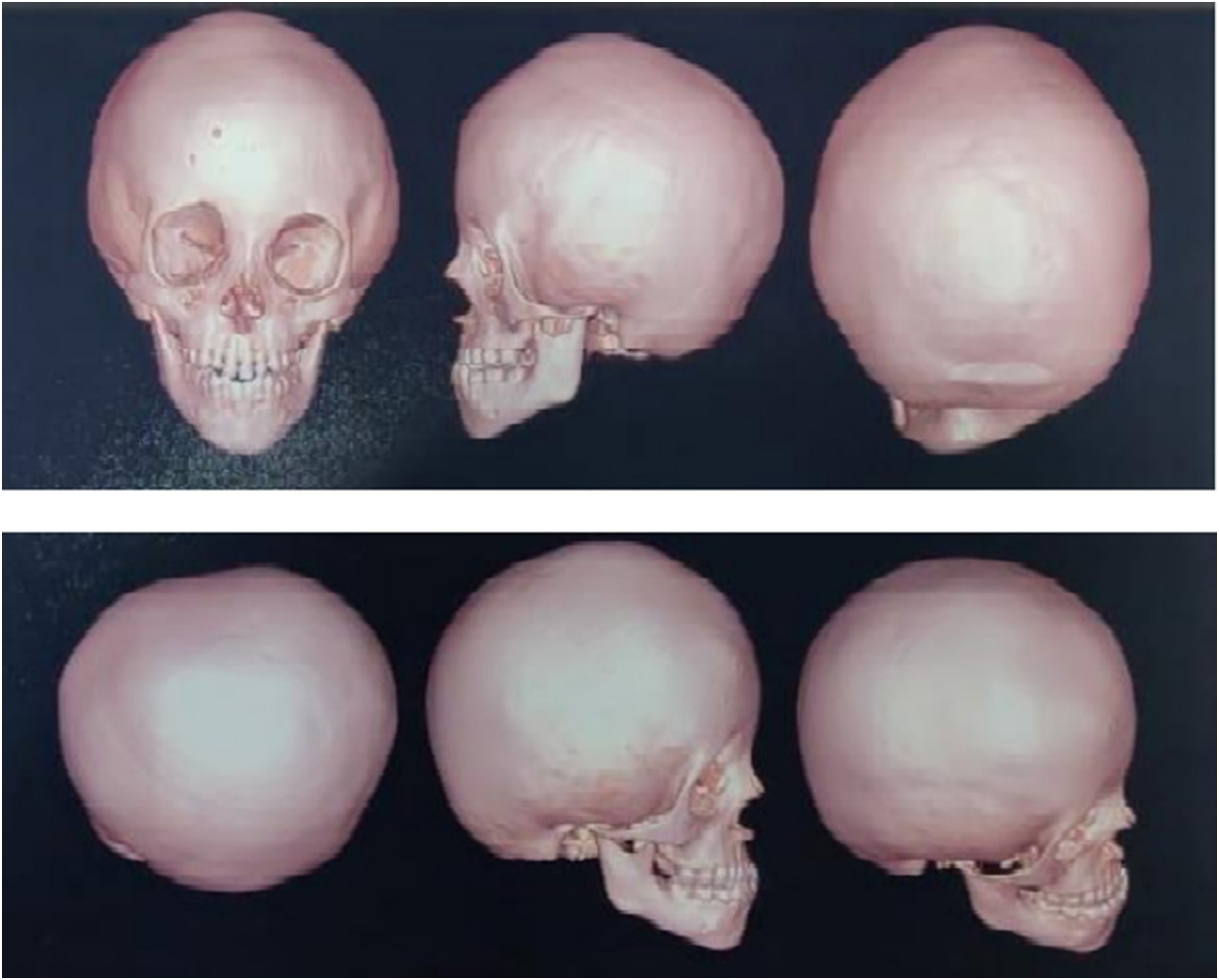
CT 3D reconstruction of skull of the female sibling.
